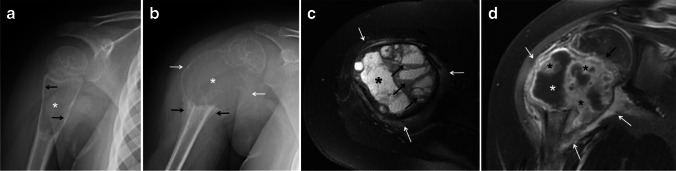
Rapid progression of a proximal humeral aneurysmal bone cyst from a type I to a type II in a 5-year-old girl. a An initial anteroposterior radiograph of the shoulder shows a proximal metadiaphyseal, lytic lesion (*asterisk*) of the humerus. The lesion causes endosteal scalloping (*arrows*), is slightly expansile and has no internal septations or matrix. b An anteroposterior radiograph of the shoulder obtained 7 months later shows significant interval growth of the lesion (*asterisk*) with blurring of the physeal margin. The lesion is now expansile and has marked cortical thinning (*white arrows*) with no sclerotic border or areas of mineralization. Aggressive periosteal reaction (*black arrows*) is identified at the distal margin of the lesion. c An axial fat-suppressed T2-weighted magnetic resonance (MR) image of the humeral lesion shows the expansile, multiseptated humeral lesion with a dominant cystic cavity (*asterisk*), fluid-fluid levels (*black arrows*) and mild adjacent soft-tissue edema (*white arrows*). d A coronal fat-suppressed, contrast-enhanced T1-weighted MR image of the humeral lesion clearly shows the focal bone expansion and physeal involvement (*black arrow*). The lesion is multicystic; the dominant cyst (*white asterisk*) displays peripheral contrast enhancement and areas of enhancing solid tissue (*black asterisks*). A rim of peripheral enhancing edema is present (*white arrows*)
